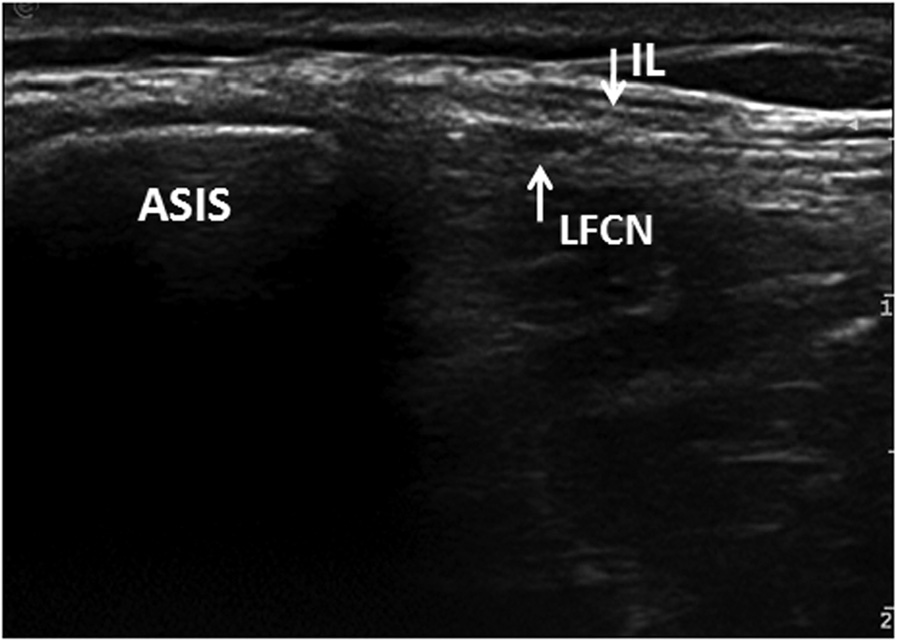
Transverse ultrasound image shows the nerve passing through the inguinal ligament. ASIS: anterior superior iliac spine; LFCN: lateral femoral cutaneous nerve; IL: inguinal ligament.
